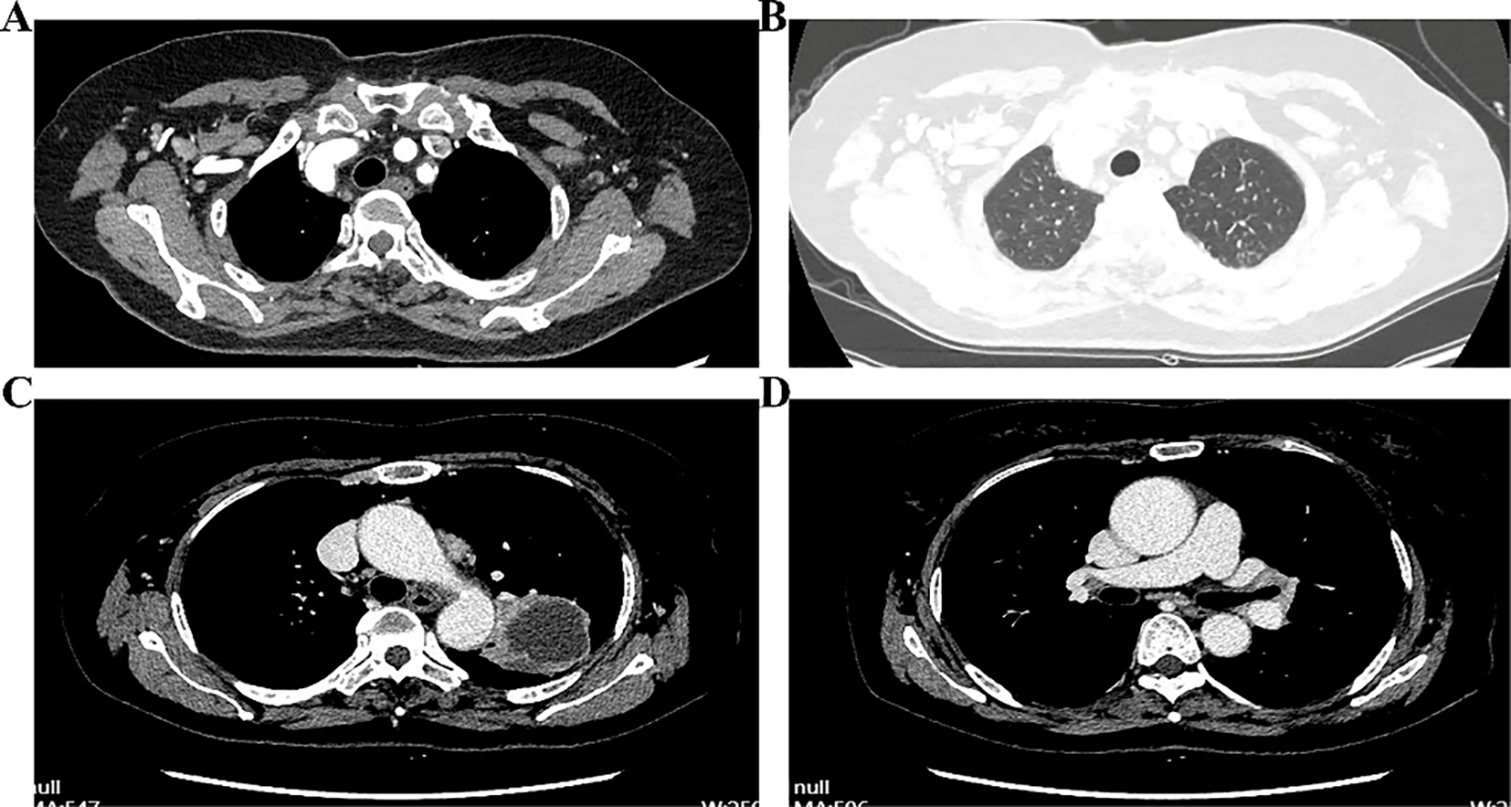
Enhanced chest CT showing the right upper lung lesion. (A, B) Ground-glass nodules in the upper right lung using lung window and mediastinal window; (C, D) Mediastinal lymph nodes using mediastinal window.
